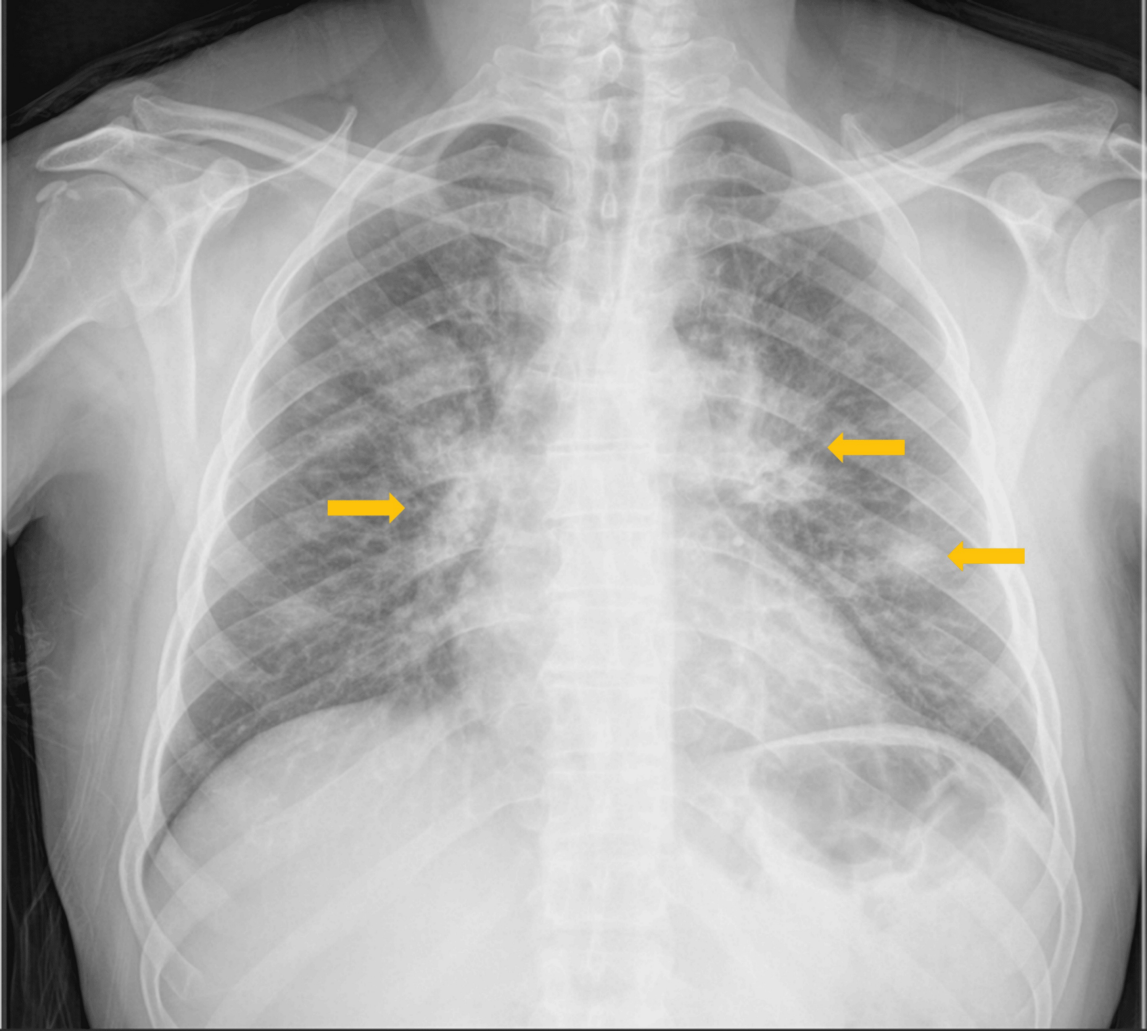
Initial chest X-ray that led to pneumonia diagnosis, showing bilateral diffuse opacities/infiltrates (yellow arrows).
